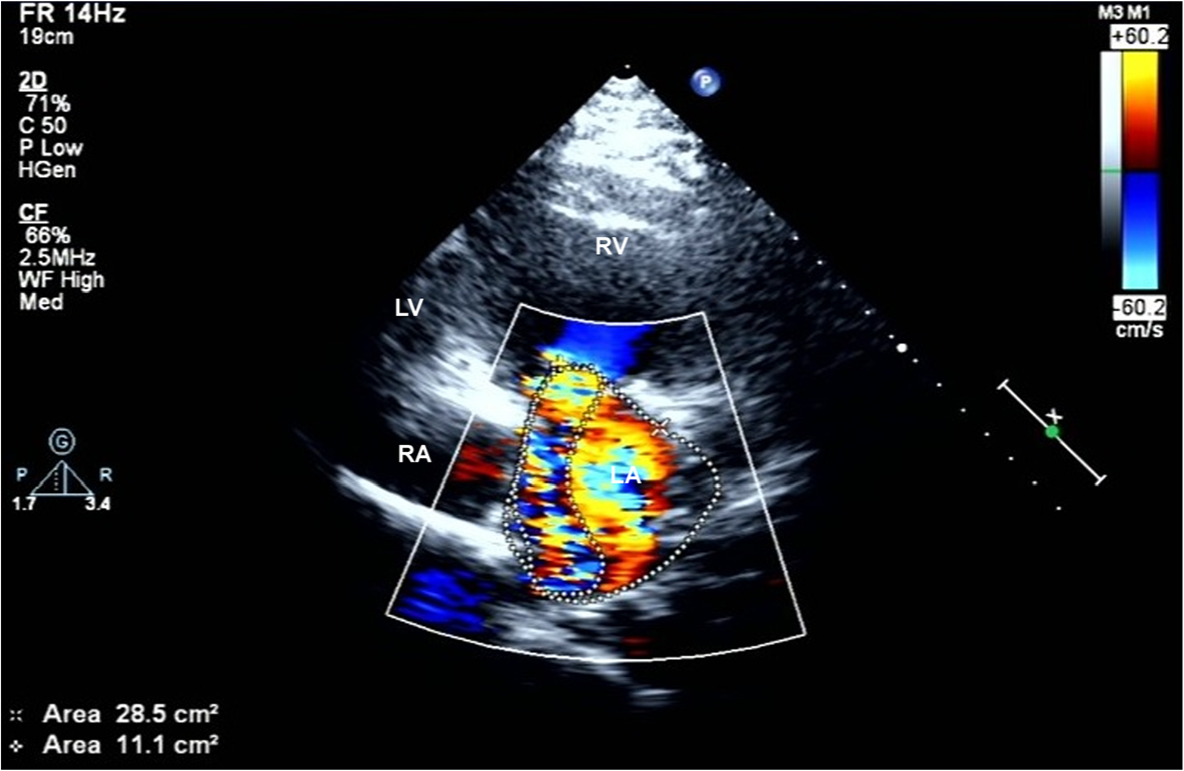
Severe tricuspid valve regurgitation jet was visible in Color Doppler mode. LA = left atrial; RA = right atrial; RV = right ventricle; LV = left ventricle
